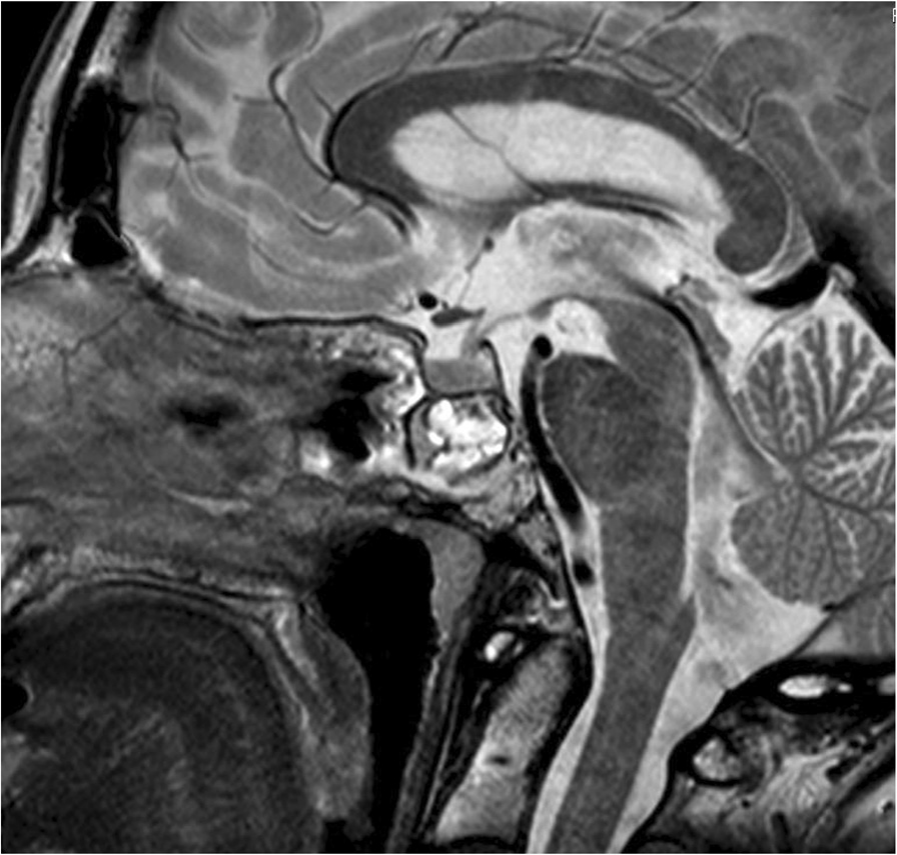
T2-weighted sagittal images showing a predominantly hyperintense lesion involving the posterior wall of the clivus just anterior to the basilar artery. The sphenoid sinus shows evidence of packing done during the first surgery with inflammatory changes.
